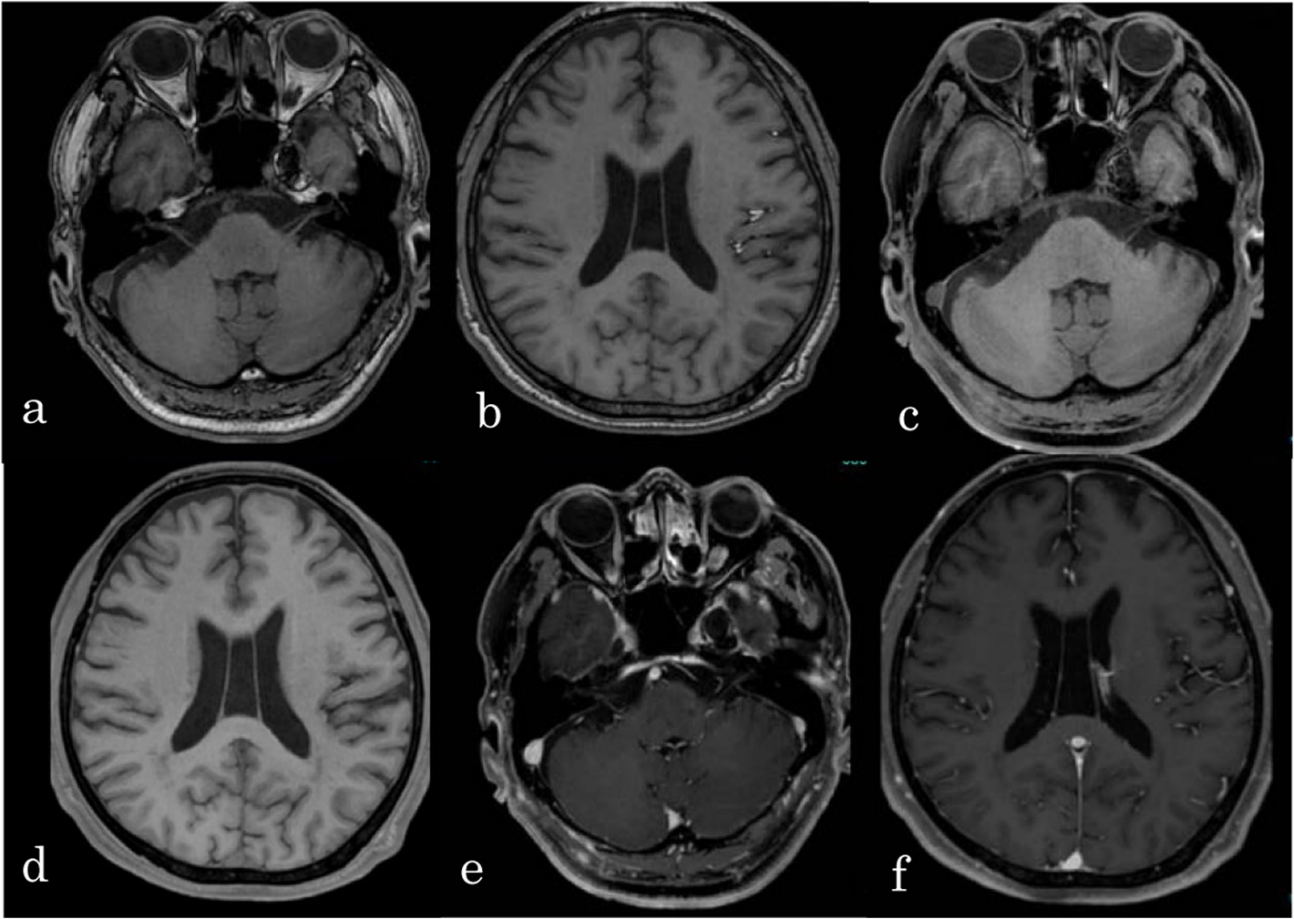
Initial magnetic resonance images of the brain. a, b T1-weighted images showing a 20 mm mass in the left cavernous sinus and fat drops in the Sylvian fissure. c, d T1-weighted fat-saturated images showing the suppression of the hyperintense drops. e, f T1-weighted contrast-enhanced images showing the lesions were not enhanced with gadolinium infusion
